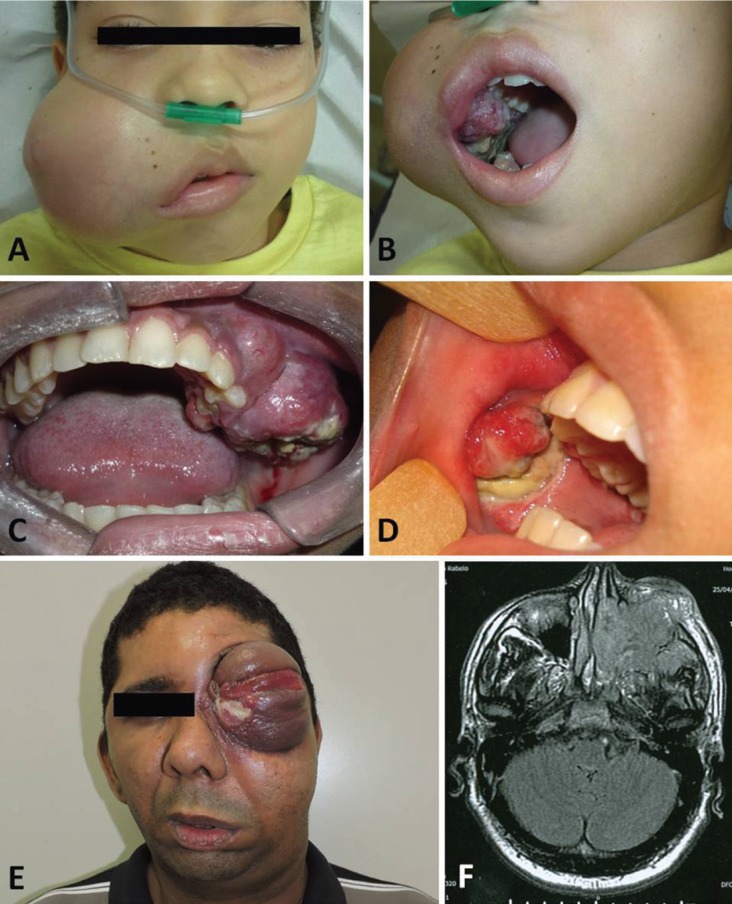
Clinical presentation of the three cases of RMS affecting the oral cavity. A) Case 1. Embryonal RMS causing a significant facial asymmetry. B) Intra-oral exam revealed a diffuse ill-defined soft-tissue neoplasm with irregular surface and areas of necrosis. C) Case 2. Embryonal RMS affecting the maxillary mucosa of a 23 year-old female patient causing a painful swelling with ulcerative regions. D) Case 3. Embryonal RHS affecting the cheek mucosa of a 10-year old female patient. Areas of necrosis are easily seen. E) Clinical presentation of one case of RMS affecting the orbit and the maxillary sinus of a 38-year-old male patient. F) Computed tomography demonstrated that left maxillary sinus was completely obliterated by the neoplasm.
